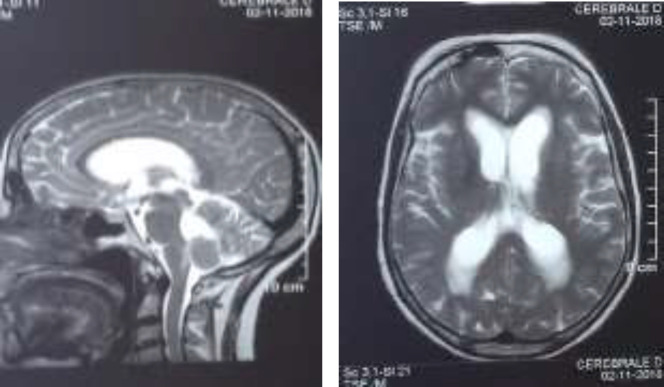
Axial (A) and Sagittal (B) sections of a brain MRI on T2-weighted imaging showing acute tri-ventricular hydrocephalus.Figure B can also notice the lesion in the 4th ventricle causing the hydrocephalus
